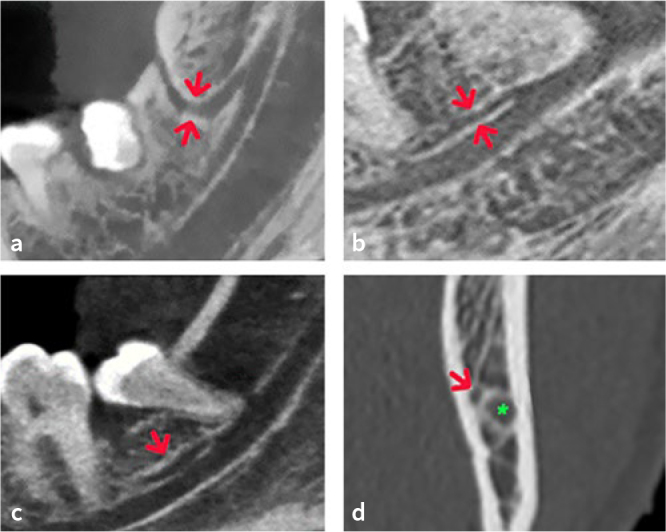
Multiplanar reconstruction CBCT images showing different types of BMCs: a. retromolar canal (red arrows); b. dental canal (red arrows); c. forward canal (red arrow); d. buccolingual canal (red arrow). Green asterisk indicates the mandibular canal.
